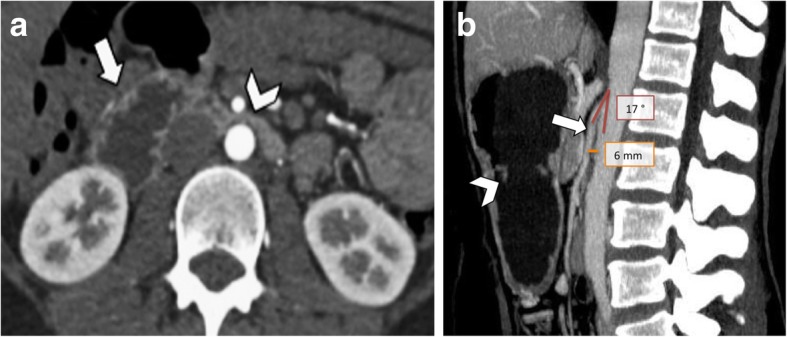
A 48-year-old woman with superior mesenteric artery syndrome. a Axial CT image on the arterial phase shows dilatation of the second portion of the duodenum (arrow), due to the compression of the third portion (arrowhead) between the abdominal aorta and the SMA. b Sagittal CT image of the abdomen shows gastric dilatation (arrowhead) caused by compression of the third portion of the duodenum between the abdominal aorta and the SMA (arrow). This sagittal reconstruction also allows measurement of aortomesenteric angle (17°) and aortomesenteric distance (6 mm), values diagnostic for mesenteric artery syndrome
